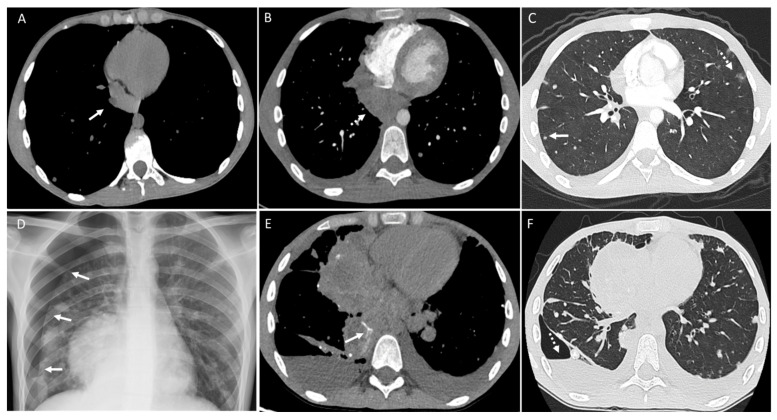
Patient with osteosarcoma and lung and pleural metastasis. Axial non-enhanced image (A) shows a right mediastinal pleura-based soft tissue lesion (arrow). Axial enhanced image (B) from a follow-up CT shows an interval increase in the size of the pleural-based soft tissue mass (dashed arrow) with a new small pleural effusion. Axial enhanced image (C) shows multiple lung nodules, including subpleural solid nodules (arrow), and nodules with a “halo sign” (dashed arrow). Frontal chest radiograph (D) from a 1-month follow-up shows interval development of right pneumothorax (arrows). Axial non-enhanced images (E,F) show a further increase in the size of the mediastinal pleura mass with interval development of intralesional calcification/ossification (arrow), left pleural effusion, and right hydropneumothorax (dashed arrow) in a subsequent follow-up exam.
